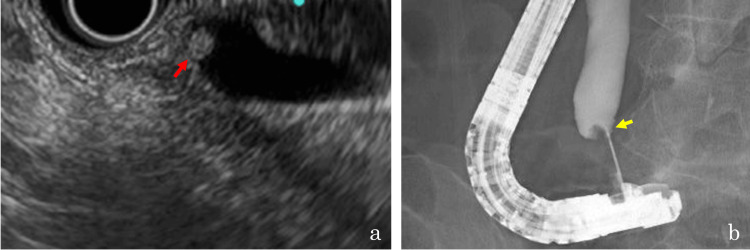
A polypoid lesion in the distal bile duct near the papilla. (a) Endoscopic ultrasonography shows a 7 mm low-echoic polypoid lesion (red arrow) in the distal bile duct near the papilla. (b) Fluoroscopy-guided forceps biopsy is performed for a polyp-like defect (yellow arrow) in the distal bile duct near the orifice of the papilla.
